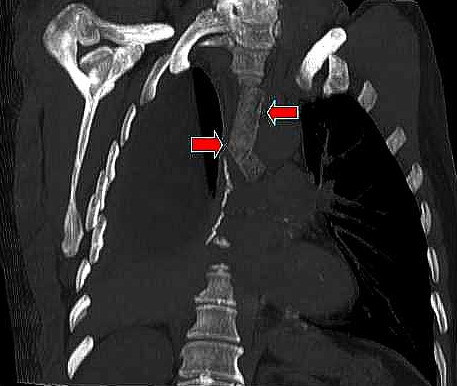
CT multiplanar reformation (MPR), oblique coronal view, with lung window clearly demonstrating patency of the left arm of the of the modified Dumon stent (red arrows).
